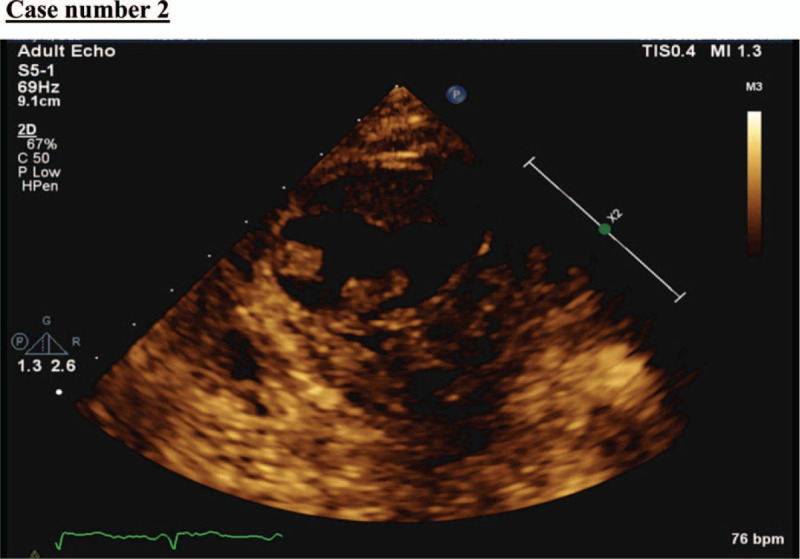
Echocardiography showed apical thrombus 5.3 × 1.7 cm in size.
